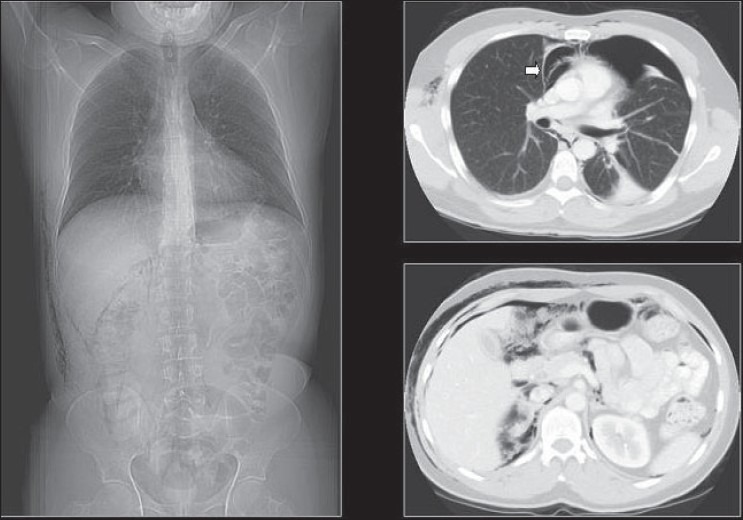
A scout film and axial CT scans showing the distribution of air following a retropneumoperitoneum. Note the pneumomediastinum (arrow)
